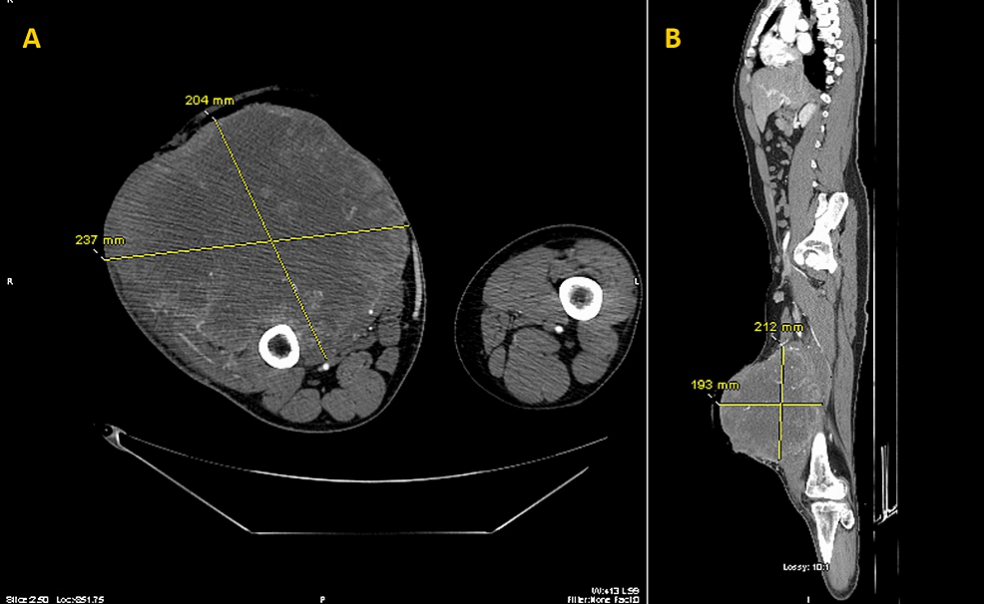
CT image of the right lower limb with fibrosarcoma protruding anteriorly from the quadriceps muscle A - Axial Plane, B - Sagittal Plane
